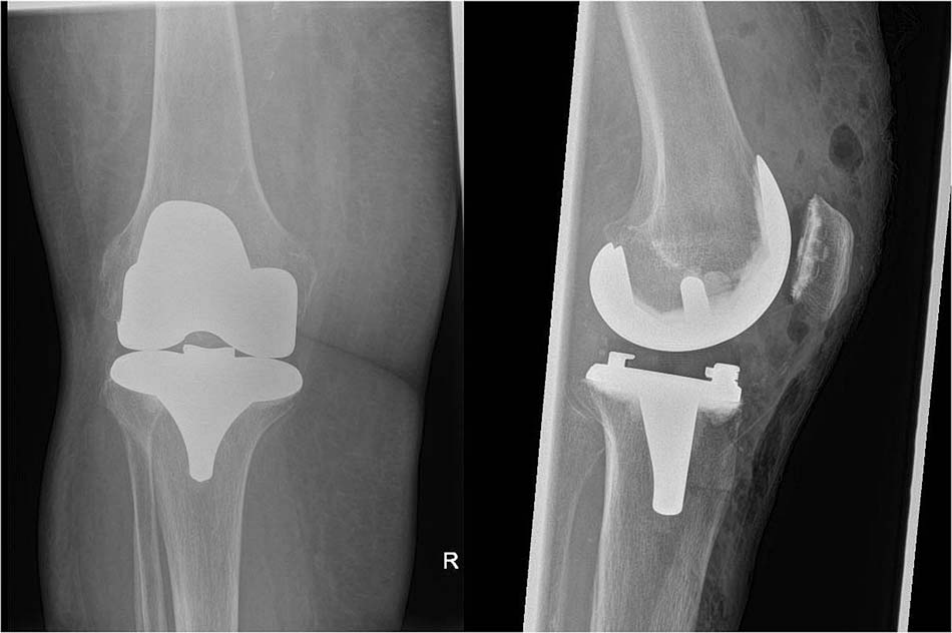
Post-operative AP and lateral X-rays of the knee.
